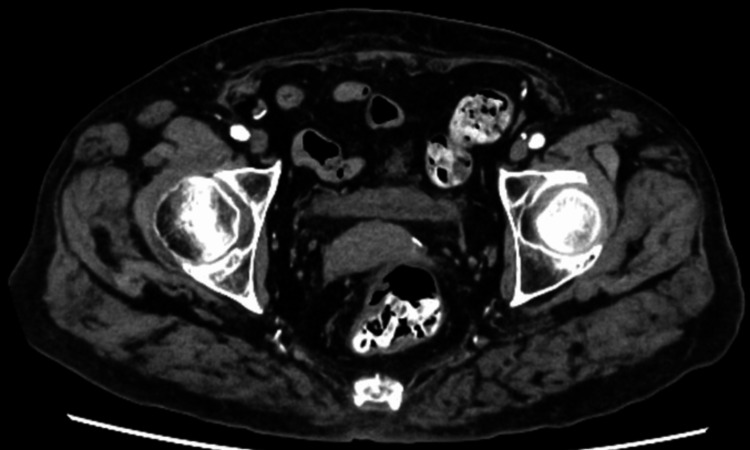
Final follow-up computed tomography scan: resolution of the femoral pseudoaneurysm Final follow-up contrast-enhanced computed tomography imaging showing resolution of the femoral pseudoaneurysm with no evidence of residual infection or inflammation.
